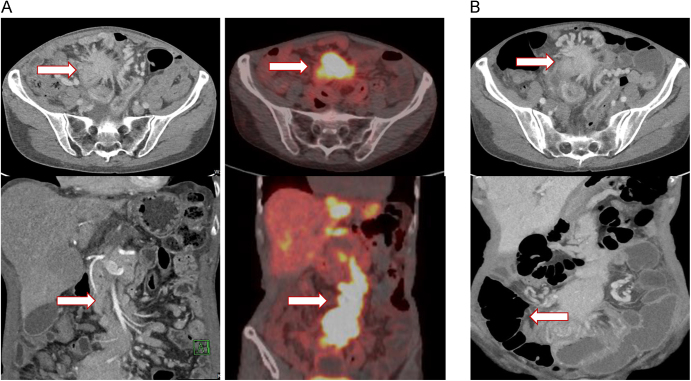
CT and ^68^Ga-DOTATATE PET scan of patient 2. (A) Pre-treatment CT scan and ^68^Ga-DOTATATE PET showing large central mesenteric mass and retroperitoneal lymphadenopathy. (B) Post-treatment CT scan showing a stable 5.6 cm mesenteric mass with chronic SMA encasement and distal occlusion of the SMV.
